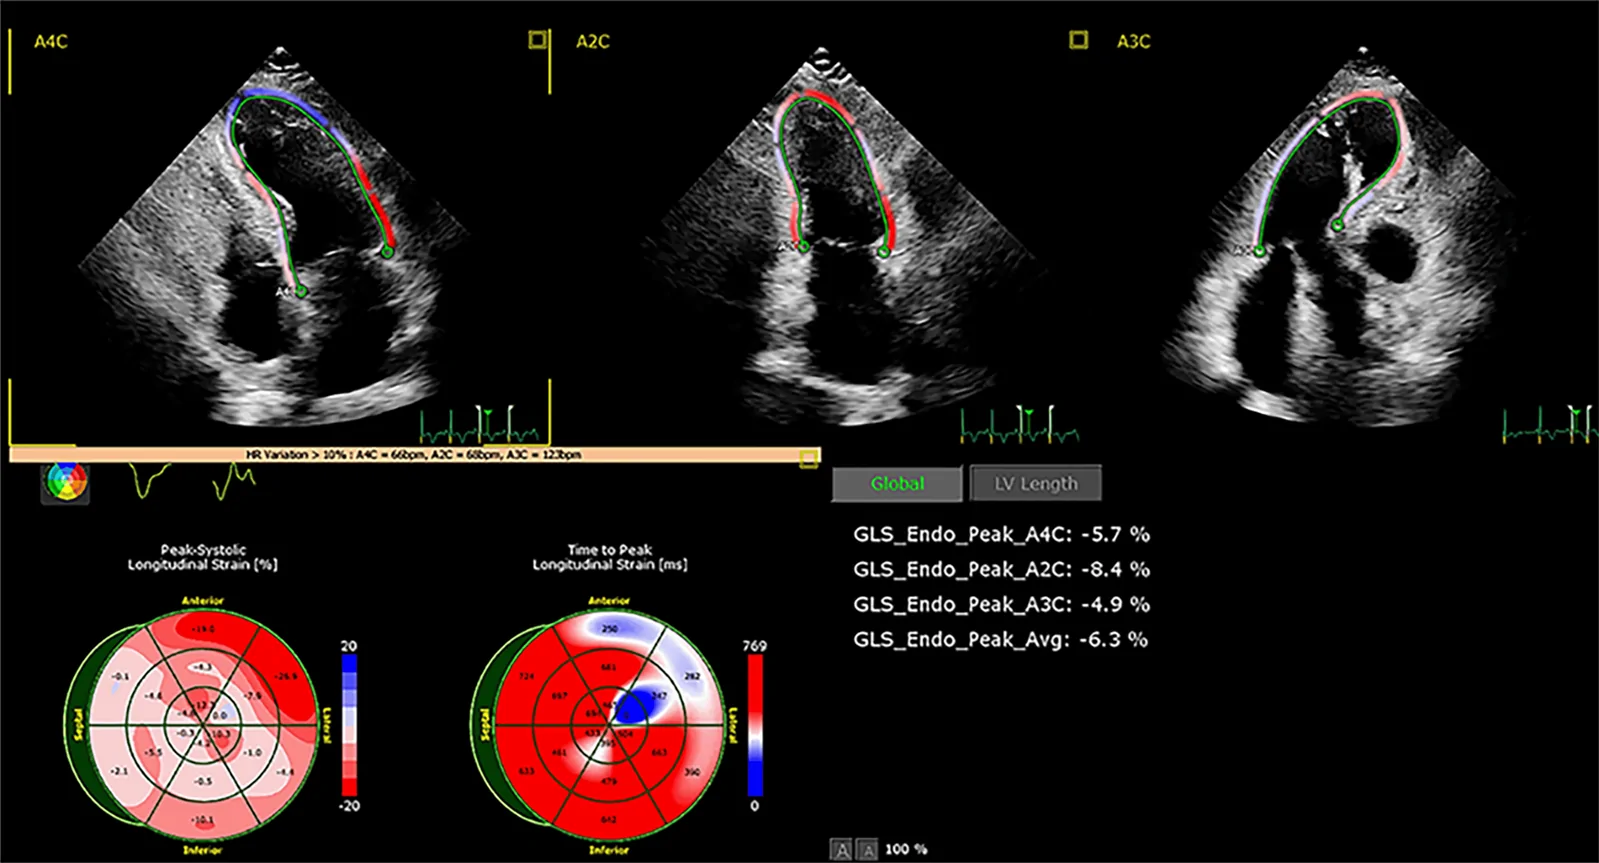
Acute myocardial strain abnormalities detected by two-dimensional speckle-tracking echocardiography (2D‑STE) Acute‑phase 2D‑STE demonstrating typical apical ballooning and severely reduced left ventricular global longitudinal strain.
